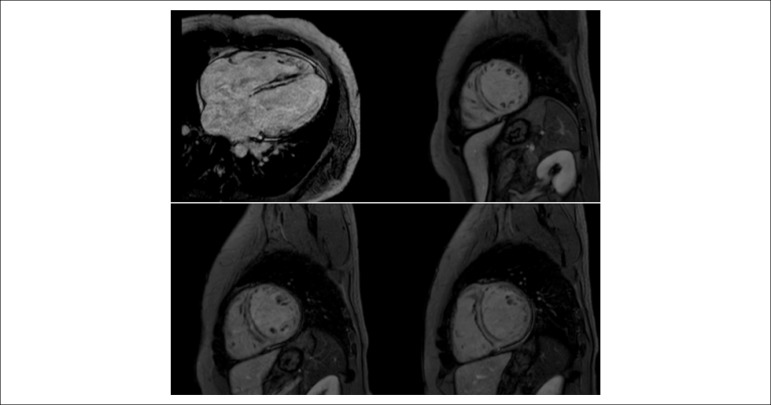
Cardiac magnetic resonance imaging showing extensive and diffuse area
